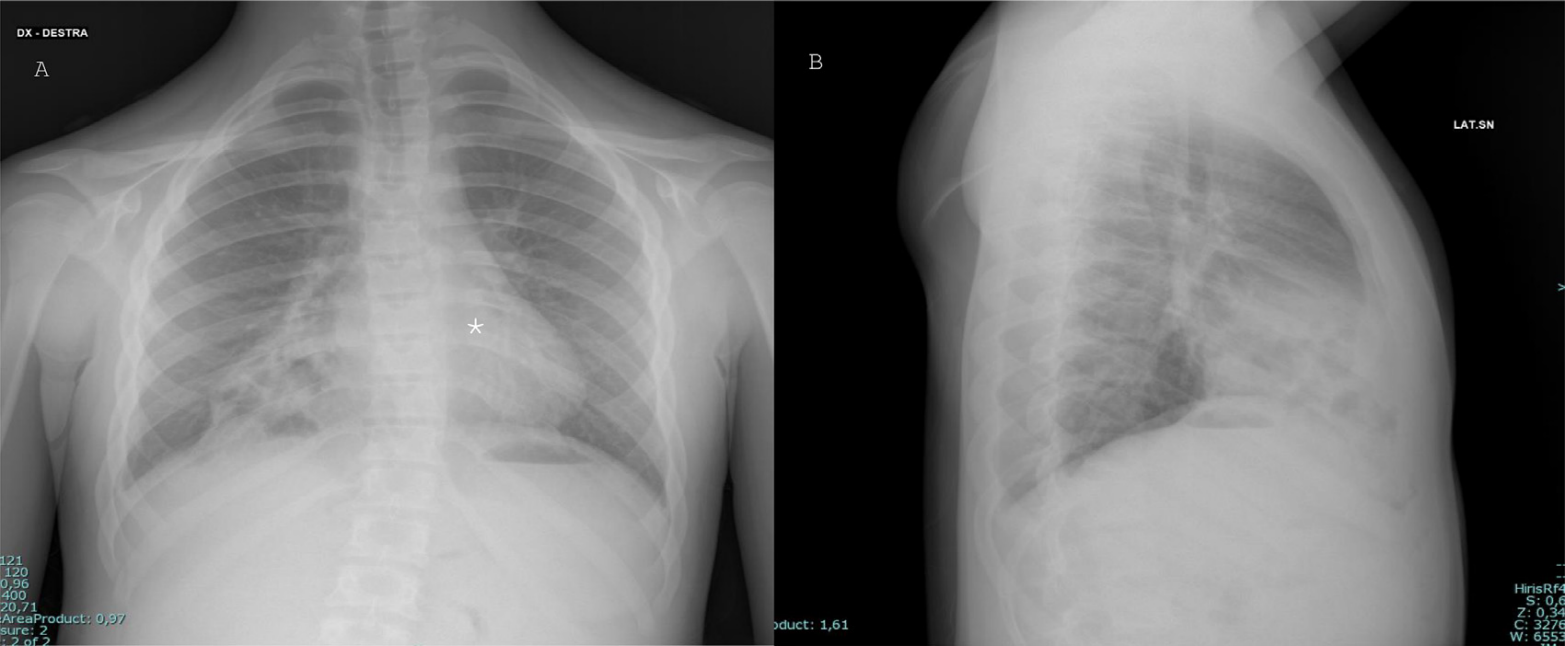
PA (A) and LL (B) projections chest x-ray showing showed multiple round areas of “bubble-like” increased transparency amidst a nonhomogeneous and blurred reduced transparency in the right basal paracardiac area (asterisk), a radiologic appearance that could be called “Bubbly heart”.
